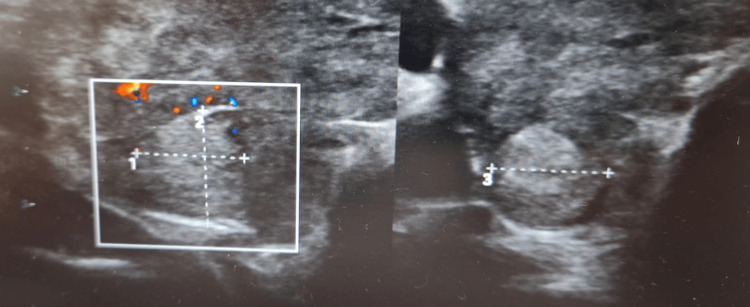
Ultrasound Thyroid Showing a 1 cm Nodule
